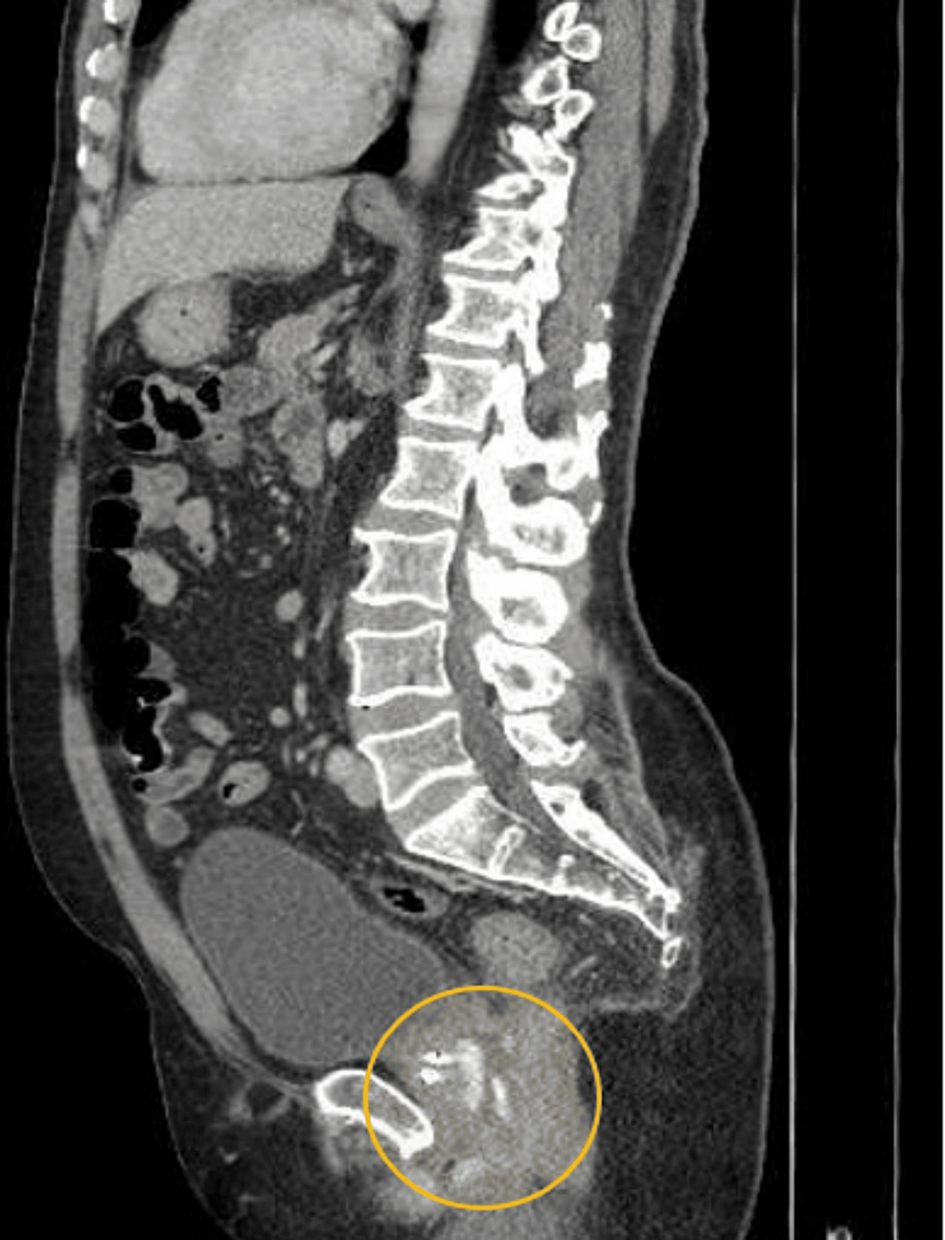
CT of the pelvis with contrast (sagittal view) Moderate soft tissue inflammation at the perineum with a 3.2 x 5.3 x 5.0 cm heterogeneous complex fluid collection (shown within the yellow circle) located between the prostate and the rectum. There are areas of hyper-density within the collection as well as a small hyper-dense focus within the perineal soft tissues. The location of the collection is consistent with hydrogel placement.
